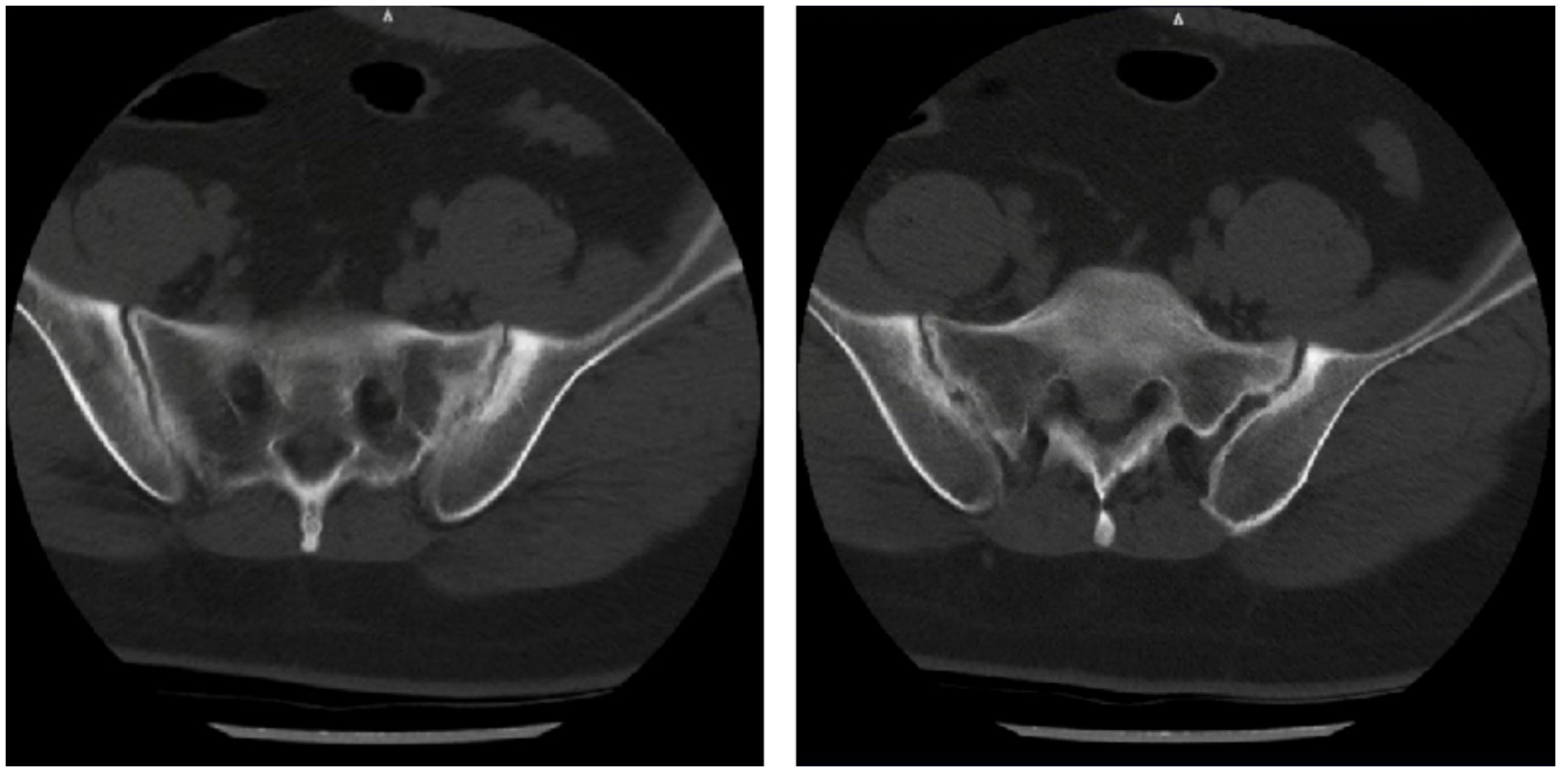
Lumbosacral spiral CT imaging: bilateral sacroiliac joints show blurred articular surfaces, serrated edges, and narrowed joint spaces, consistent with ankylosing spondylitis.
